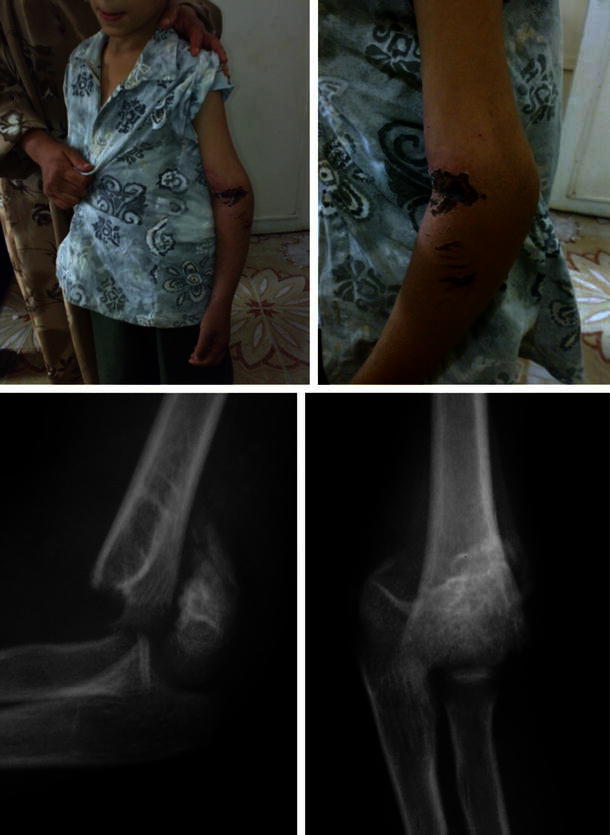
(*Top*) clinical photograph showing elbow swelling, deformity with sagging and crusts in a case of extension supracondylar fracture humerus neglected for 27 days. (*Bottom*) X-ray showing off-ending of the fragments with marked posterior displacement with early callus formation. Nerve conduction study showed incomplete radial nerve involvement (patient 1)
